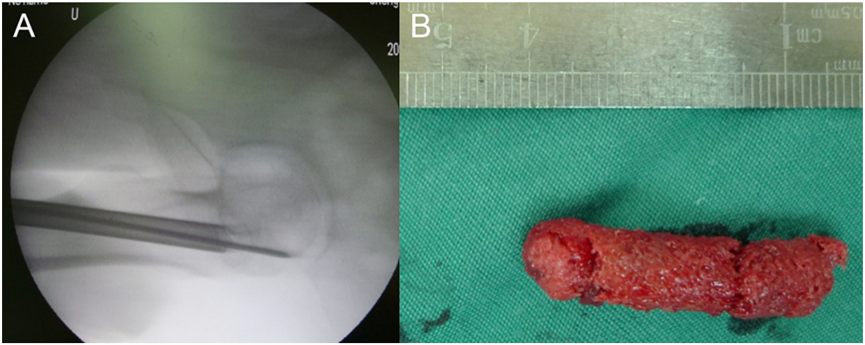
Image of operation procedure. (A) Radiography of the femoral head at the time of the operation. The Kirschner wire and 10-mm diameter trephine were driven into the mid-line of the trochanter by a cannulated drill under C-arm x-ray guidance toward the necrotic area. (B) A 3.5-cm long cylindrical bone was taken from the trochanter through the trephine and used for bone graft to enriched bone marrow incorporation.
